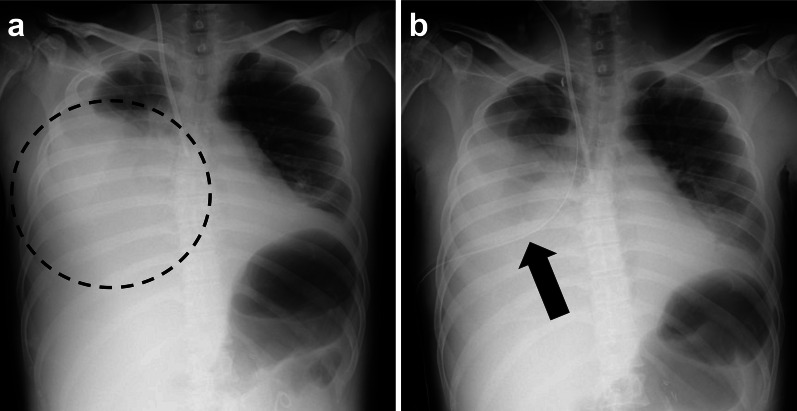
Chest radiograph images. a Chest radiograph captured on postoperative day 4. Large pleural effusion is visible within the area delineated by the dotted line. b After thoracic drain placement; the black arrow indicates the drain
